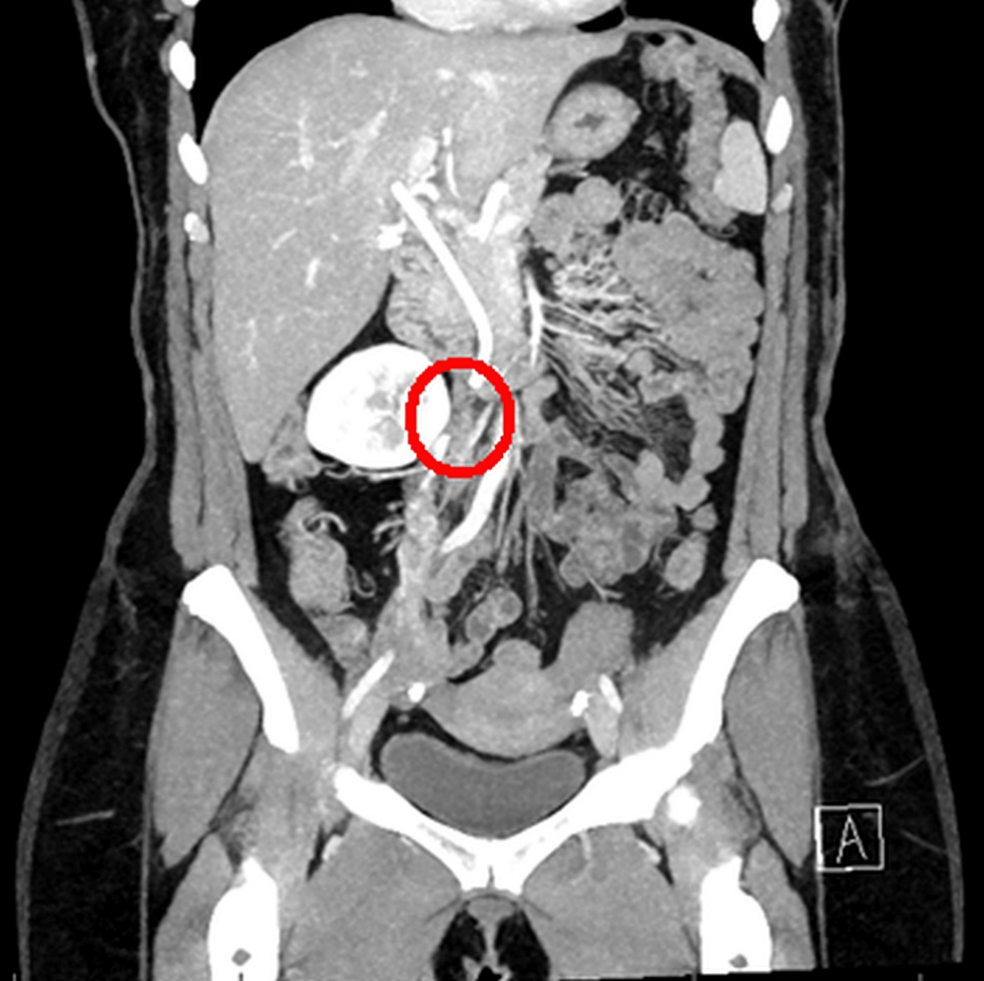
CT scan of the abdomen and pelvis with intravenous contrast showing right ovarian vein thrombosis in the red circle.
